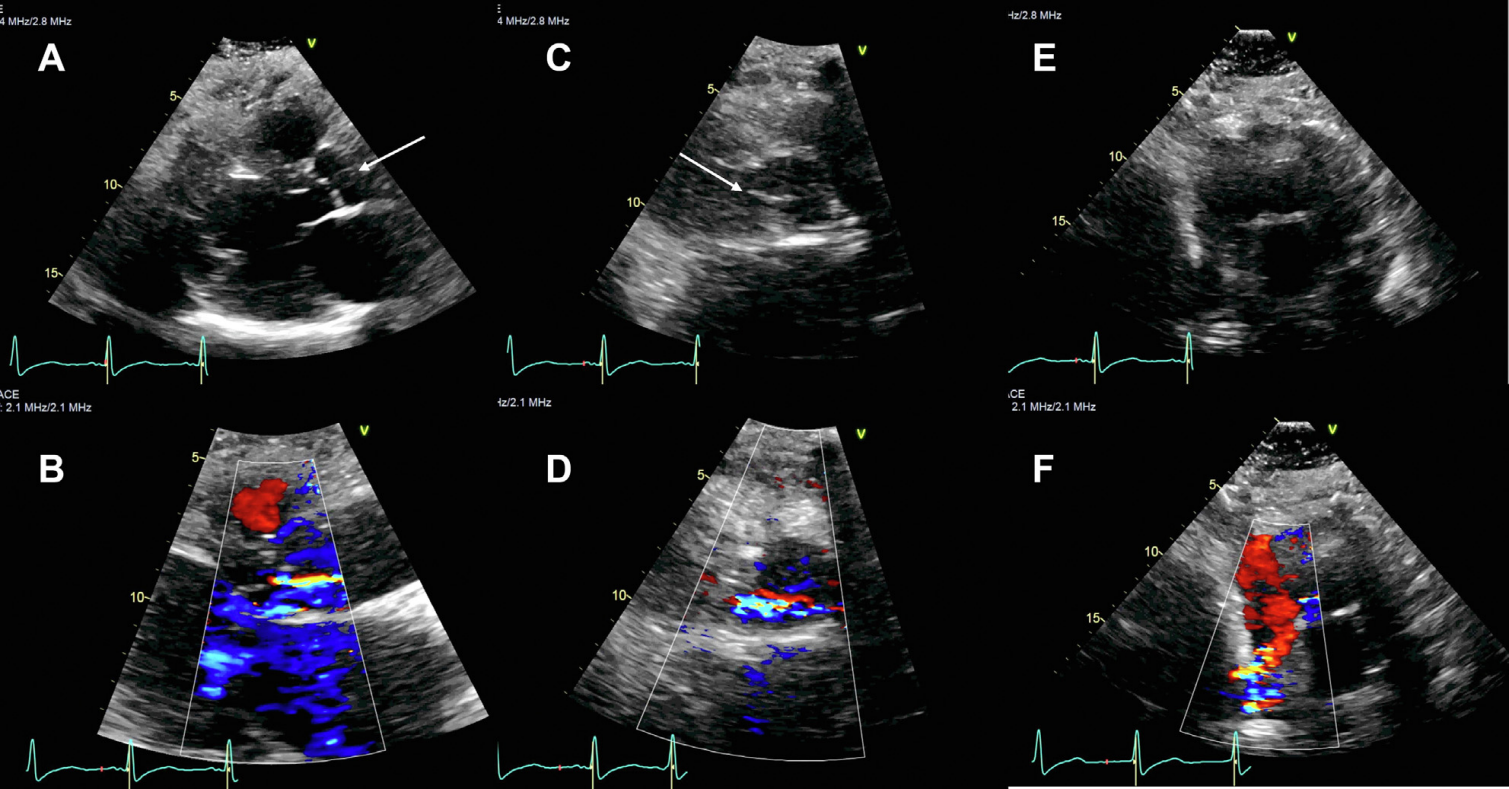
Two-dimensional TTE (performed 13 days after ICA), diastolic images without (*top row*) and with (*bottom row*) color-flow Doppler, demonstrated an unexplained echodensity (*arrows*) between the noncoronary cusp and RCC that appeared to be attached to the AV, best seen in the parasternal long axis (A) and parasternal short-axis (C) views. The associated AR in these views was reported as mild (B, D), originated between the noncoronary cusp and RCC, and was eccentrically directed toward the RCC. Apical views were suboptimal (E), but mild eccentric AR was noted (F).
